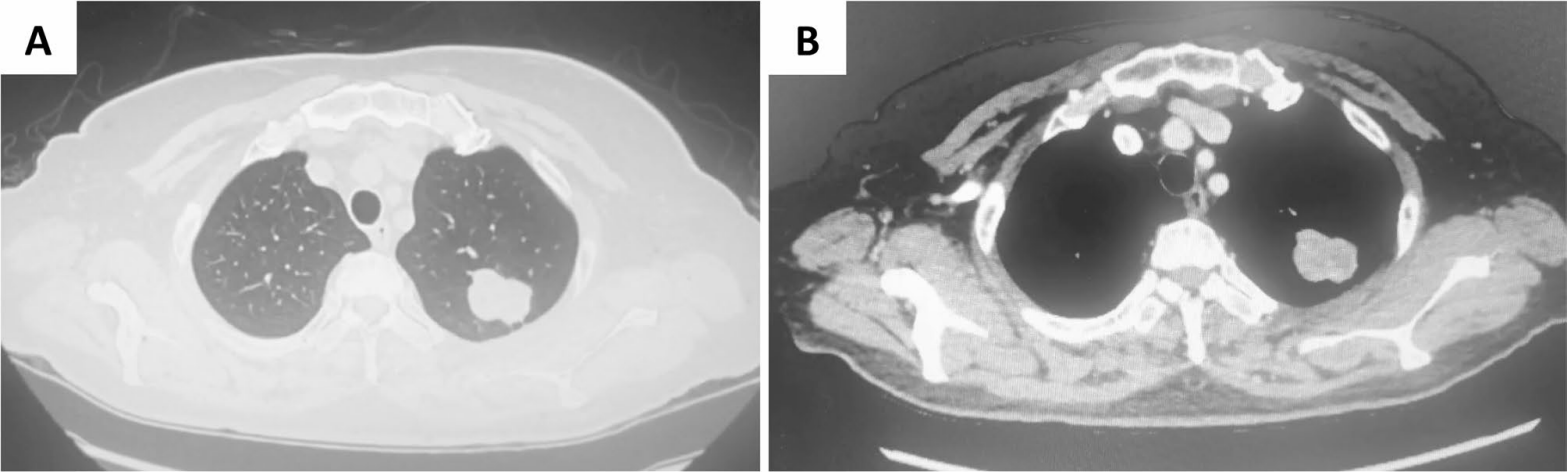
The CT scans of an inflammatory myofibroblastic tumor. A Lung window of CT shows a tumor measuring 3.2 cm in diameter in the apicoposterior segment of the left upper lobe, demonstrating a lobulated contour with peripheral spiculated margins. B Mediastinal window of chest CT shows no abnormal masses identified within the mediastinum. Both hila appear normal and pleural effusions are absent. Heterogeneous mild enhancement of the tumor is noted following contrast administration
